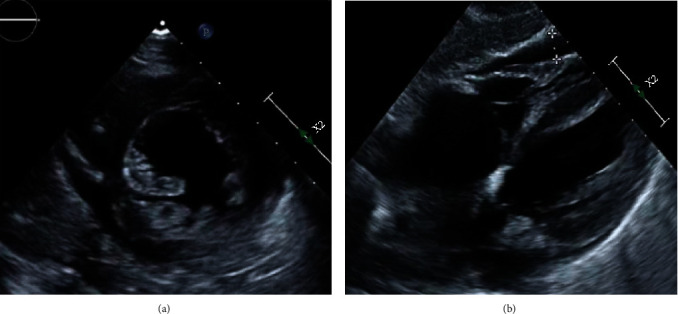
TTE showing a free-flowing pericardial effusion identified circumferentially to the heart. Mild decrease of her left ventricular systolic function, from 50% in January 2020 to 40-45%. (a) Parasternal short axis view. (b) Subxiphoid view.
